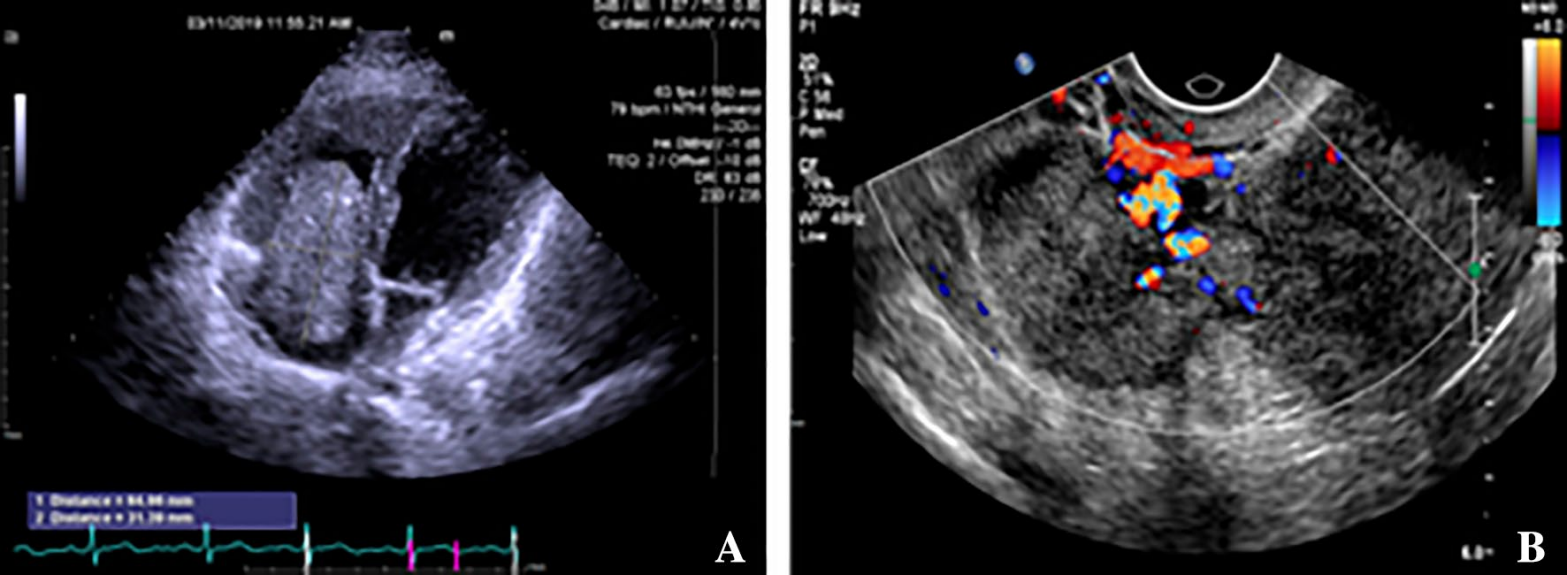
Preoperative ultrasound of Case 8. A Abnormal echo mass in the right heart cavity. B Tortuous paracervix vessels
